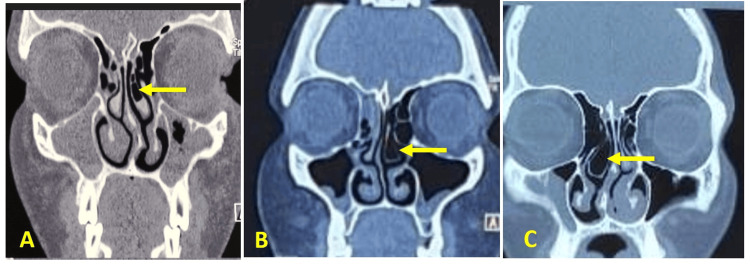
Coronal CT images of paranasal sinus showing variation in middle turbinate pneumatization A: lamellar type; B: bulbous type; C: extensive type (concha bullosa) CT: computed tomography
